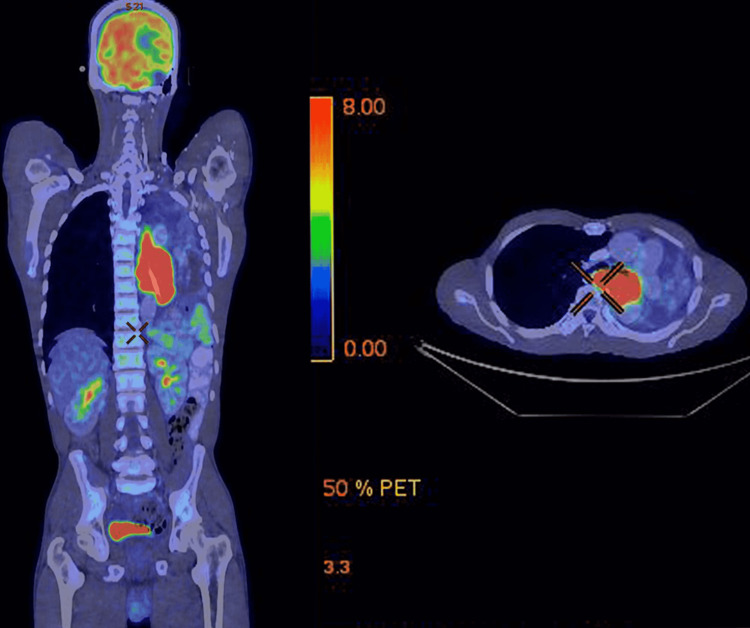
Pre-treatment PET-CT scan showing localized, unresectable lower thoracic esophageal mass
